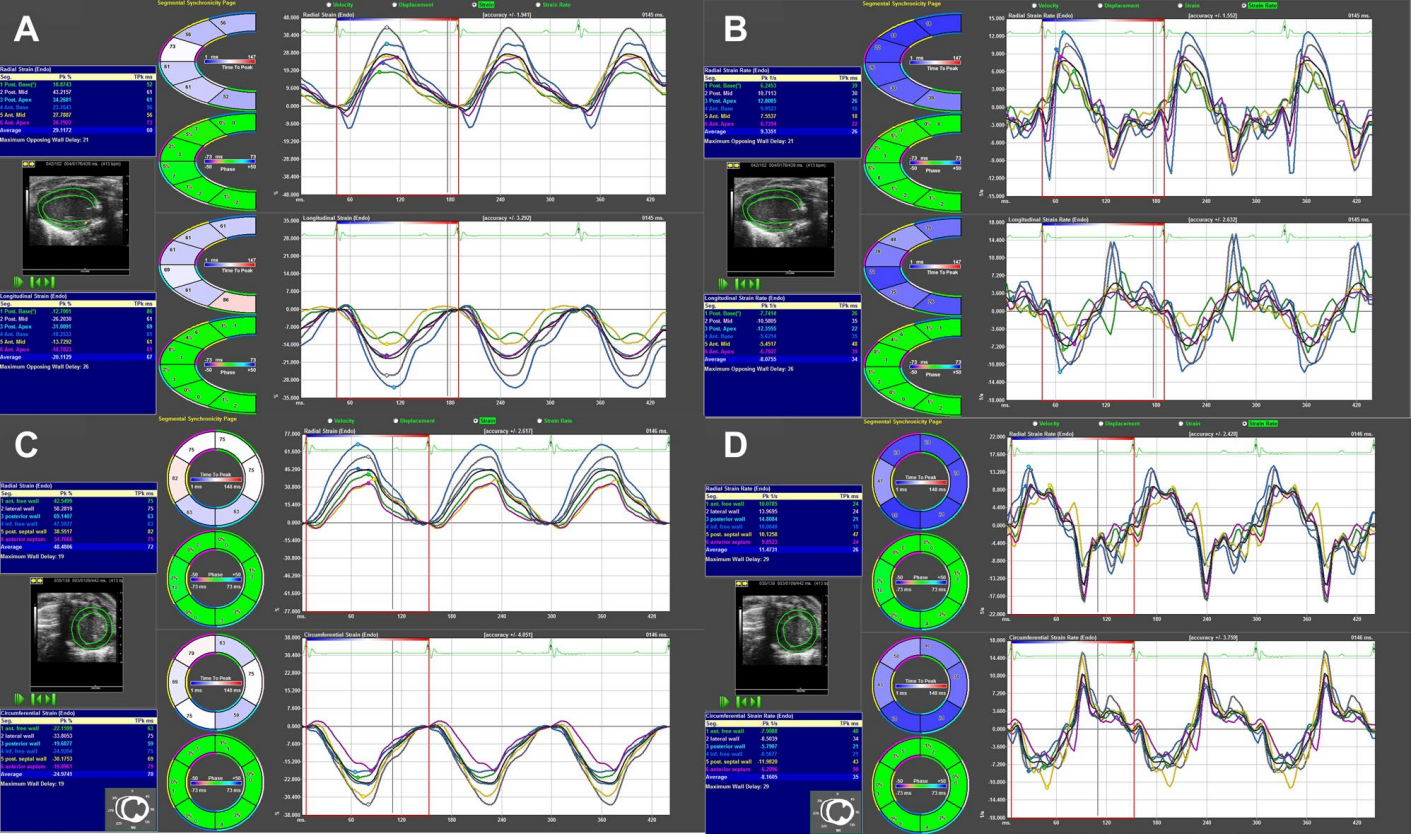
Global and regional strain and strain rate analyses in parasternal long-axis and short-axis view. (A) GLS and RLS analyses in parasternal long-axis view. (B) GLSR and RLSR analyses in parasternal long-axis view. (C) GCS, RCS, GRS, and RRS analyses in parasternal short-axis view. (D) GCSR, GRSR, RCSR, RRSR analyses in parasternal short-axis view. GLS* global longitudinal strain; *GLSR* global longitudinal strain rate; *RLS* regional longitudinal strain; *RLSR* regional longitudinal strain rate; *GCS* global circumferential strain; *GCSR* global circumferential strain rate; *RCS* regional circumferential strain; *RCSR* regional circumferential strain rate; *GRS* global radial strain; *GRSR* global radial strain rate; *RRS* regional radial strain; *RRSR* regional radial strain rate.
